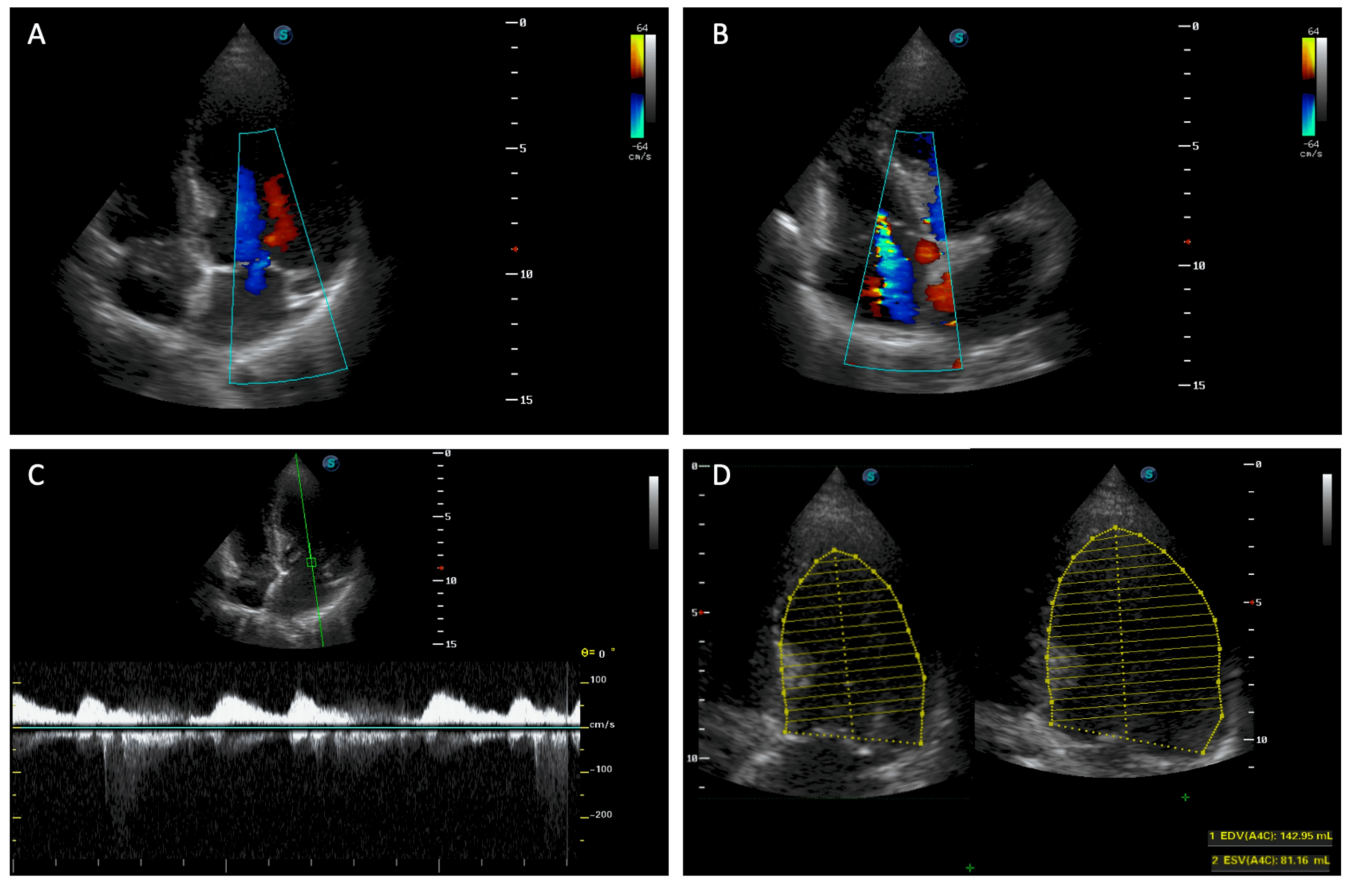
Transthoracic echocardiogram of a patient with antiphospholipid syndrome diagnosed with acute myocardial infarction showing an apical four-chamber view with color Doppler over mitral and tricuspid valves, reveling mild mitral regurgitation (A) and moderate tricuspid regurgitation (B), spectral Doppler flow over the mitral valve (C), and calculation of left ventricle systolic function using modified Simpson technique (D)
